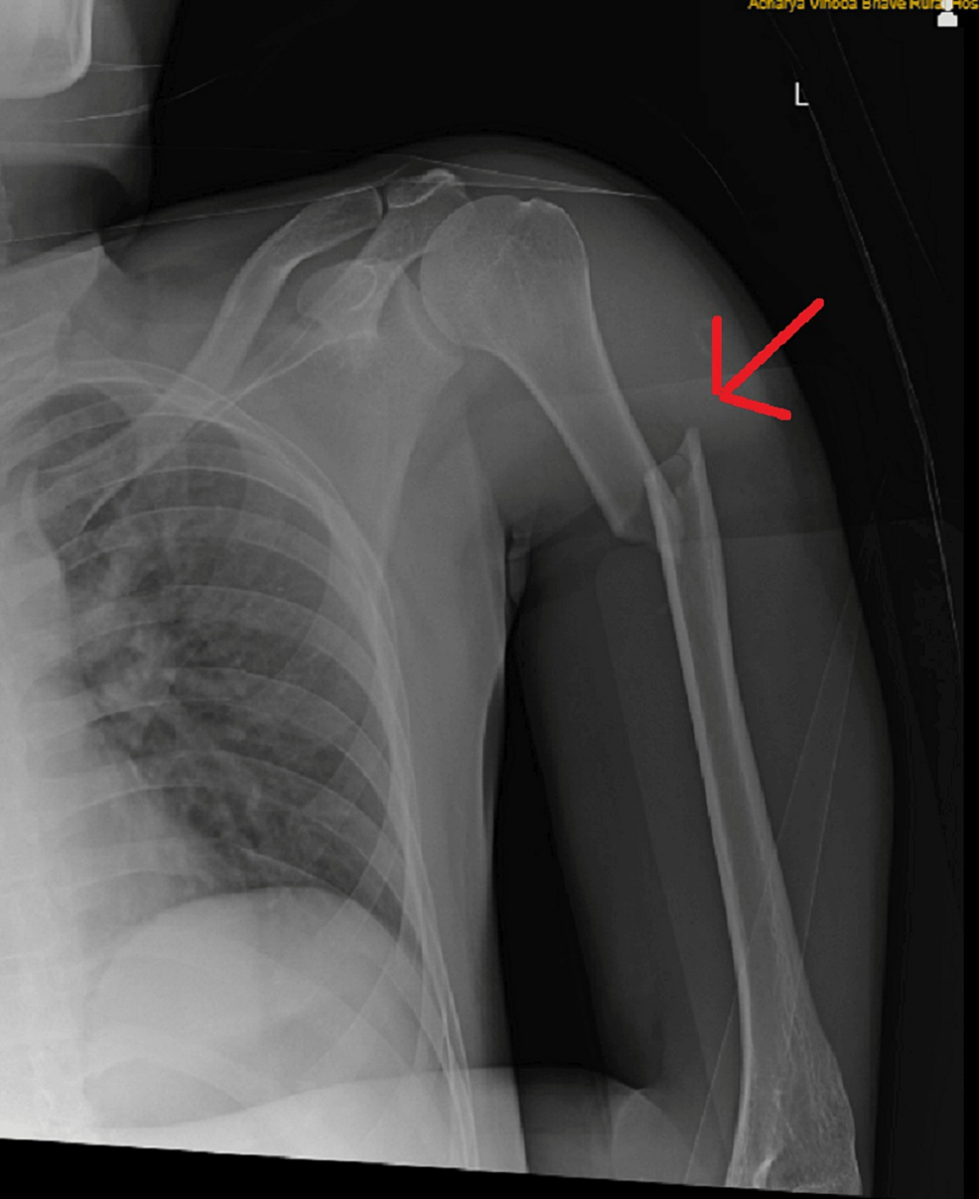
Lateral radiograph showing short oblique fracture in proximal humeral shaft region on left side
